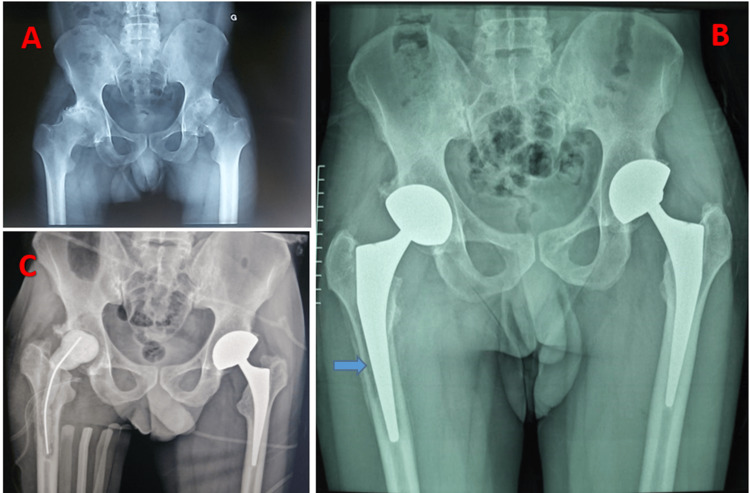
Radiographic findings in the patient in case 5 with biologic cement reaction shock A: Front pelvis radiograph showing bilateral coxarthritis at the ankylosis stage. B: Radiograph of the front pelvis showing radiographic signs (blue arrow) of septic loosening. C: Post-operative control pelvis radiograph after placement of biologic cement.
